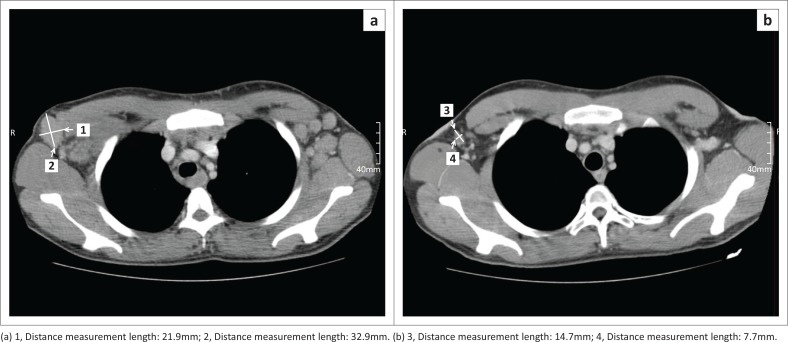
Hodgkin’s lymphoma, with the initial computed tomography (CT) (a) and restaging CT (b) Complete response to therapy with the axillary nodes regressing to normal size.
